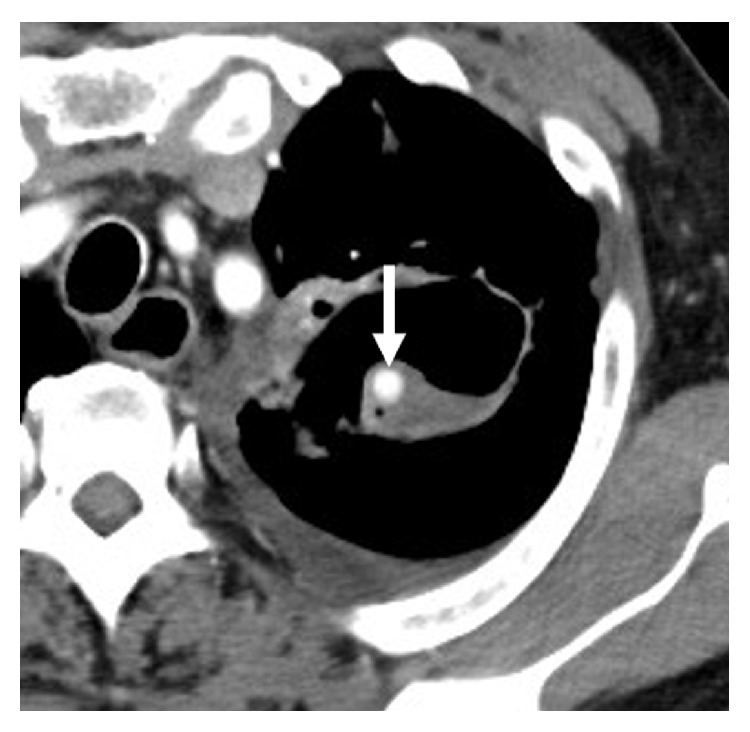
Contrast-enhanced axial computed tomography image. There is a 7 mm round aneurysm (arrow) within a cavitary lesion in the left upper lobe of the lung.
